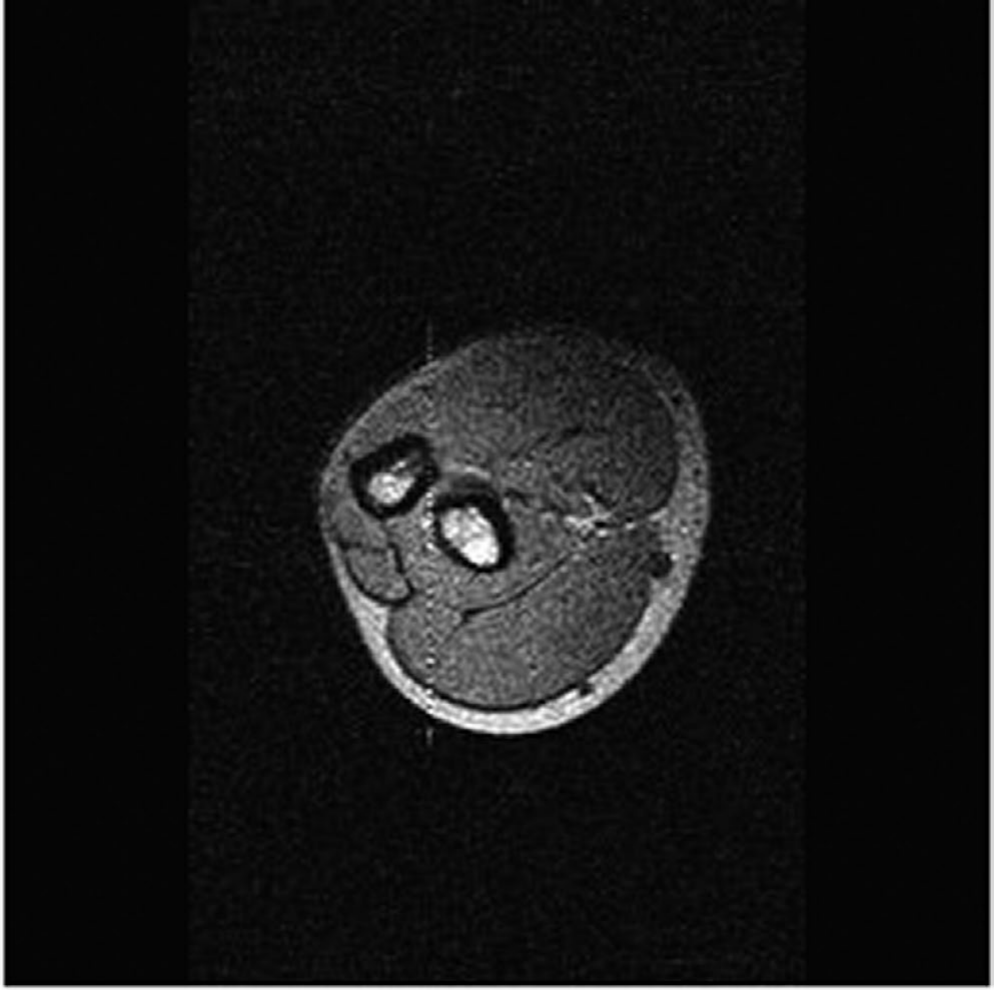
MRI without contrast, T1 image, axial view
